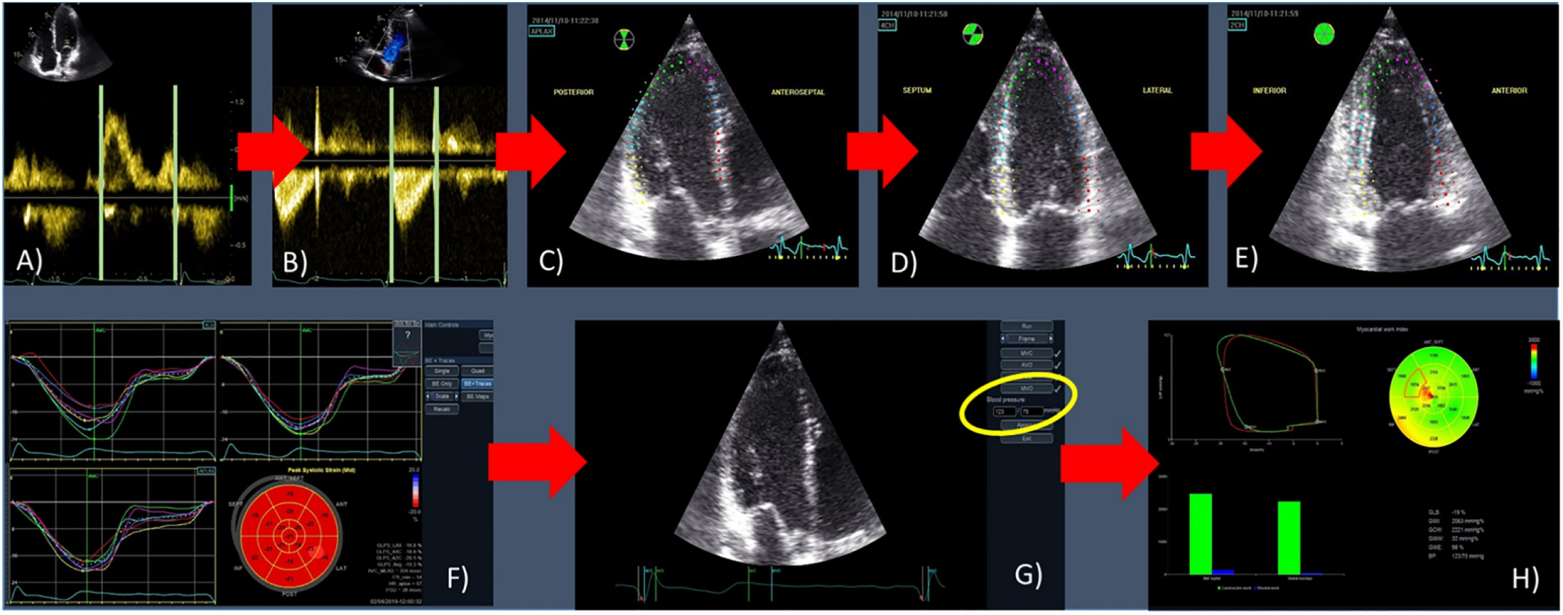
Determination of *myocardial work*. For exact timing, mitral valve opening and closure are determined using a pulsed-wave Doppler recording of the mitral inflow (A). Aortic valve opening and closure are determined using a continuous-wave Doppler recording through the aortic valve (B). Time points might be adjusted in the apical three chamber view (D), if necessary. Using *automated function imaging*, global longitudinal strain is determined in the apical three (C), four (D), and two (E) chamber views. The automated speckle tracking contour can be adjusted manually. After completion of strain determination (F), systolic and diastolic blood pressure levels have to entered (G) in order to determine global and segmental *myocardial work* (H).
